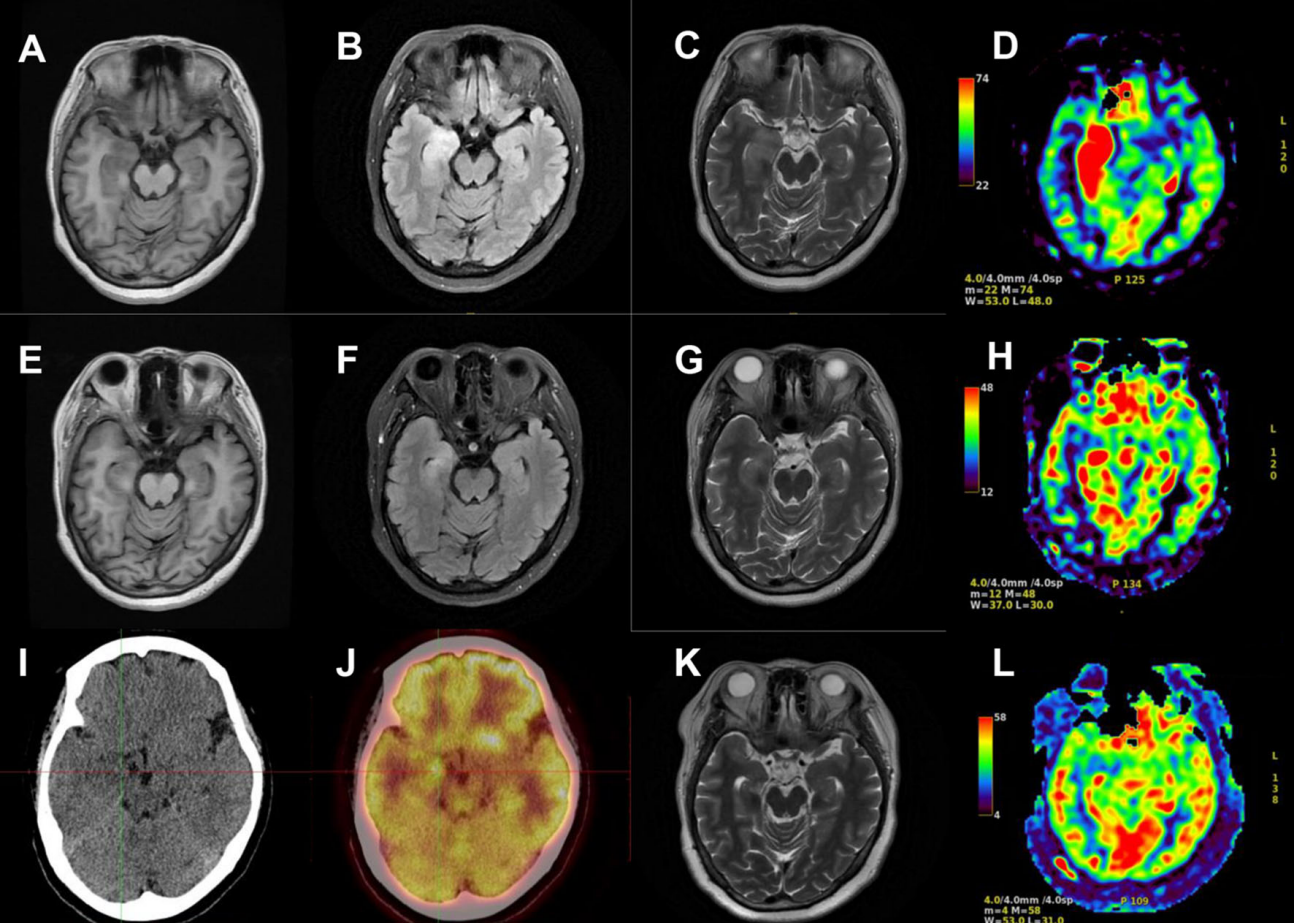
Cranial MRI and PET-CT results of the patient. Cranial MRI revealed abnormal signals in both temporal lobes on T1-weighted images (A), T2 FLAIR images (B), T2-weighted images (C), and arterial spin labeling (ASL) (D) on the second day of the first hospitalization. The extent of deep temporal lobe signals decreased bilaterally at the time of discharge (E–H). PET-CT scan indicated increased metabolism in the right medial temporal lobe (I, J). Cranial MRI revealed no obvious abnormalities on T2-weighted images (K) and ASL (L) during the second hospitalization.
